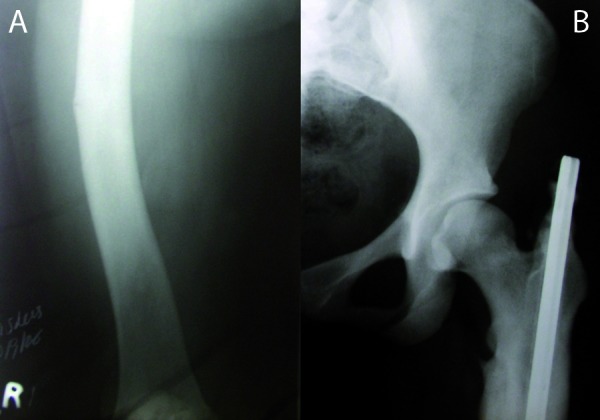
A. Long bone radiographs showed increased density of pelvic bone with a previous fracture site of left femur. B. Right femur showed lateral bowing with a fracture along the lateral aspect at the mid region.
